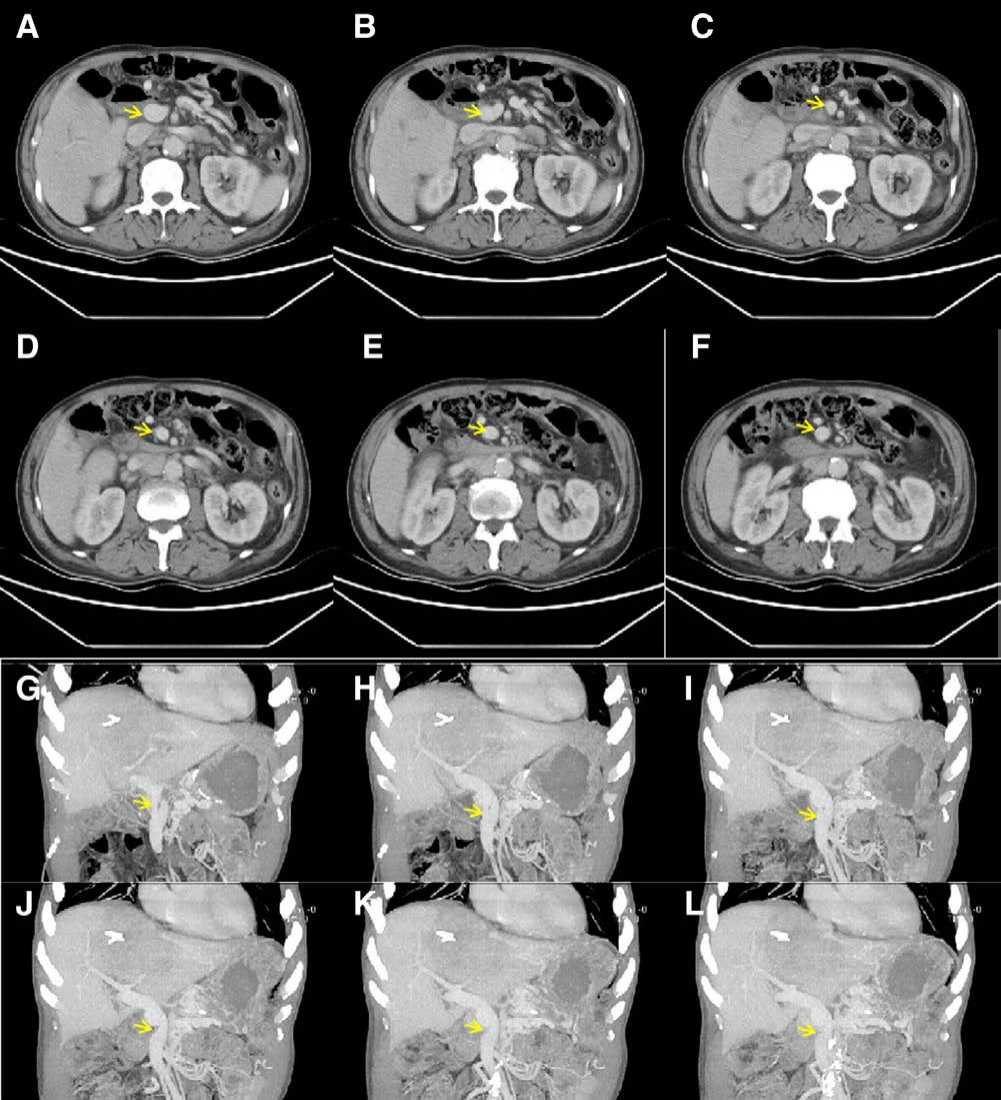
Enhanced CT performed on January 19, 2023. (A–F) Axial enhanced CT image, with the yellow arrow indicating the portal superior mesenteric vein. (G–L) Coronal enhanced CT image, with the yellow arrow indicating the ligamentum teres hepatis graft. CT = computed tomography.
